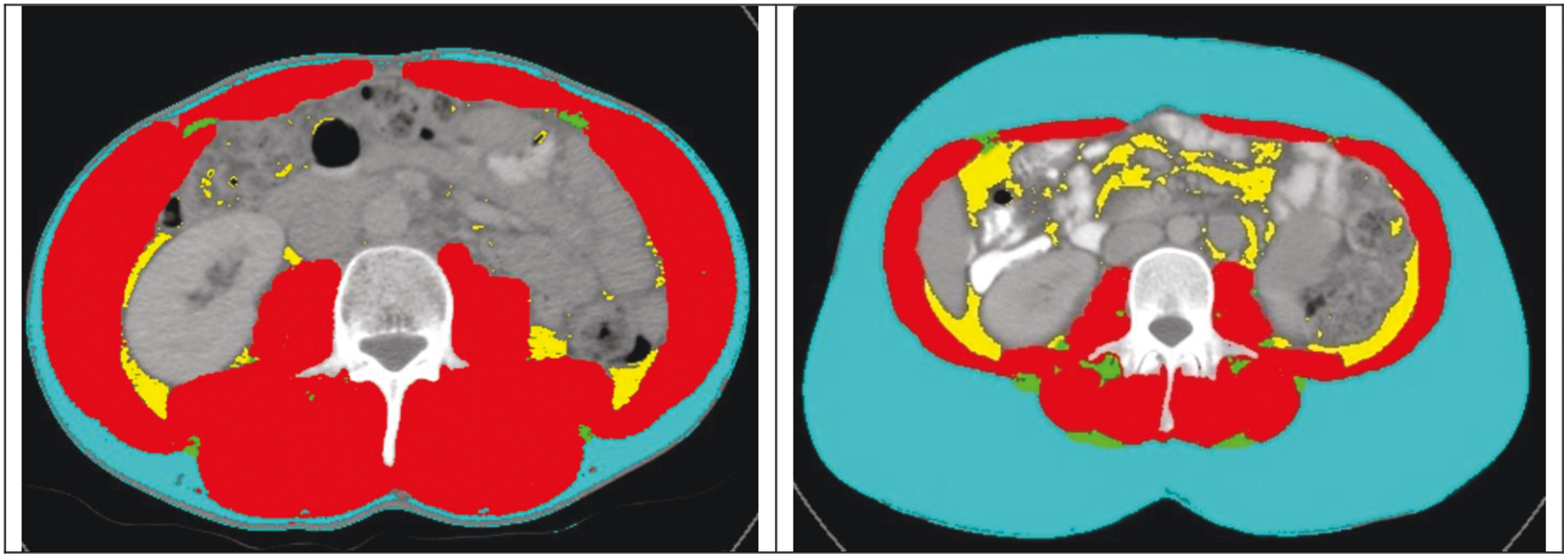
Axial CT images at the L3 level from 2 different patients with SliceOmatic (Tomovision) body composition analysis. Color overlay demonstrates subcutaneous fat area (blue), visceral fat area (yellow), intramuscular adipose tissue (green), and muscle attenuation (red) muscle (red), visceral fat (yellow), and IMAT (green).
